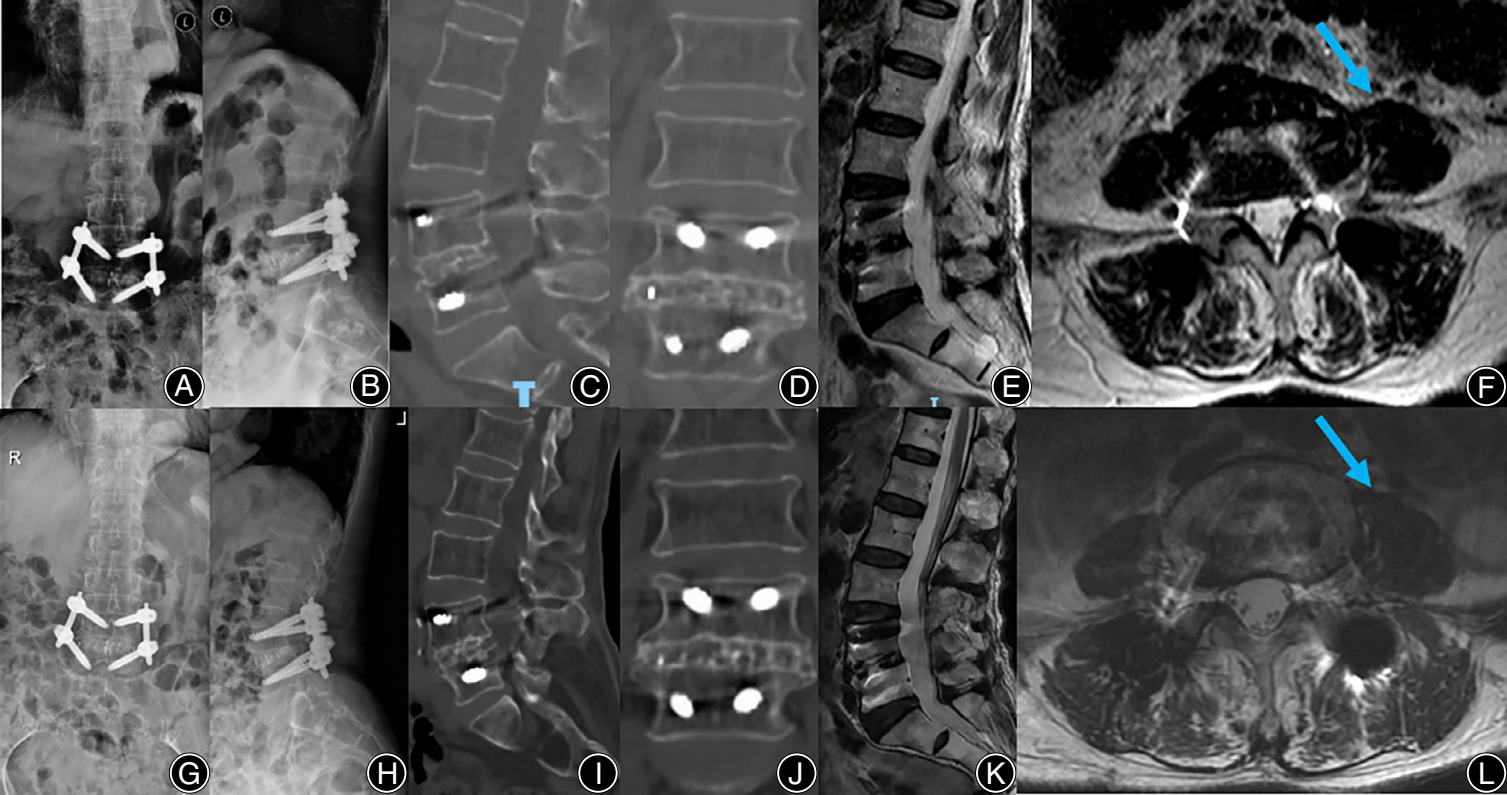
Follow‐up of the typical case. (A–F) Lumbar X‐ray, CT, and MRI at 1‐year follow‐up; (G–L) Lumbar X‐ray, CT, and MRI at 2‐year follow‐up. All follow‐up data showed solid fusion with no cage subsidence or displacement at L4‐5. Notably, compared with immediately post operation, swelling of the left psoas major reduced significantly (arrow), and its area recovered after 1‐year follow‐up.
